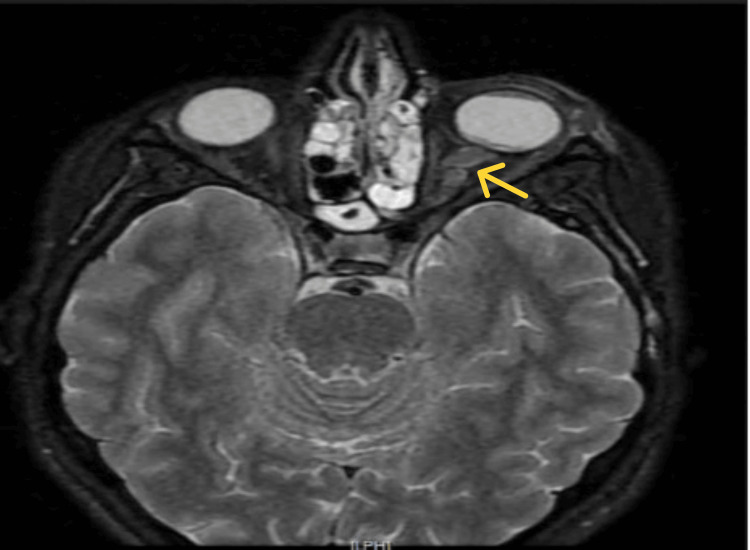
Brain and orbital MRI: tortuous and thickened appearance of the optic nerve (yellow arrow)
